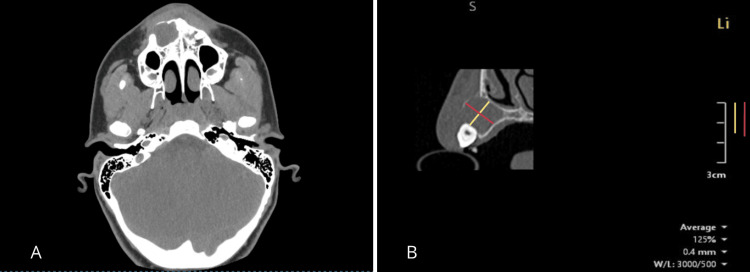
Cone beam computed tomography (CBCT) images A) Initial CBCT provided by the patient. The axial view shows the significant site of the lesion with cortical plate perforation. B) CBCT sagittal view with scale in cm. The lesion measures approximately 1.5 x 1.5 cm.
